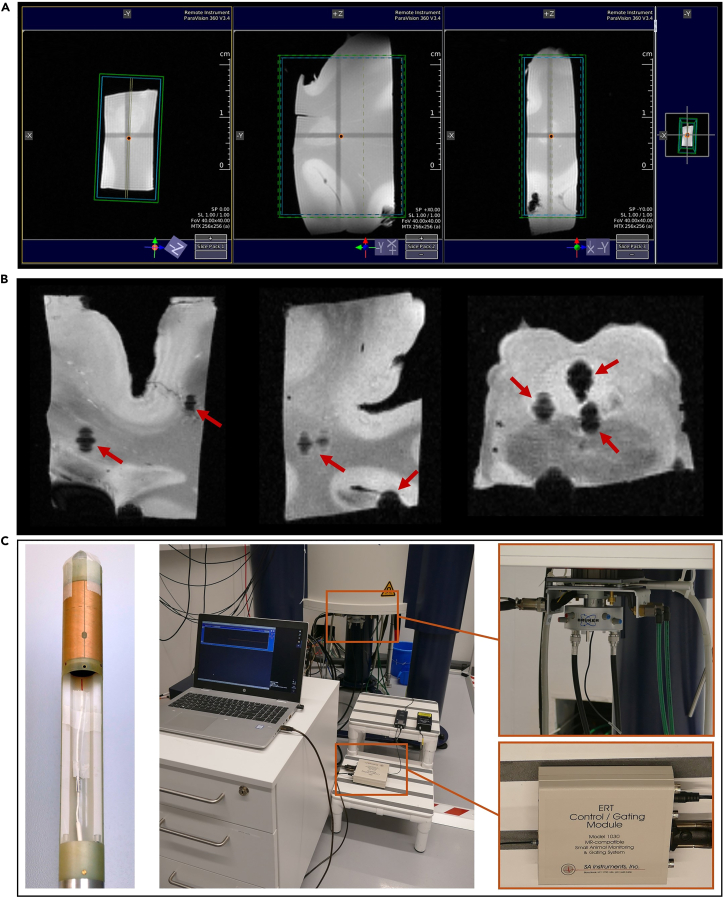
Further details on the MRI measurements setup (A) Indicative shimming volume setting that includes all meaningful regions of the sample. (B) GRE magnitude images presenting examples of persistent air bubbles trapped within tissue compartments, creating sources of artifacts. (C) Temperature monitoring setup and hardware used in our work.
